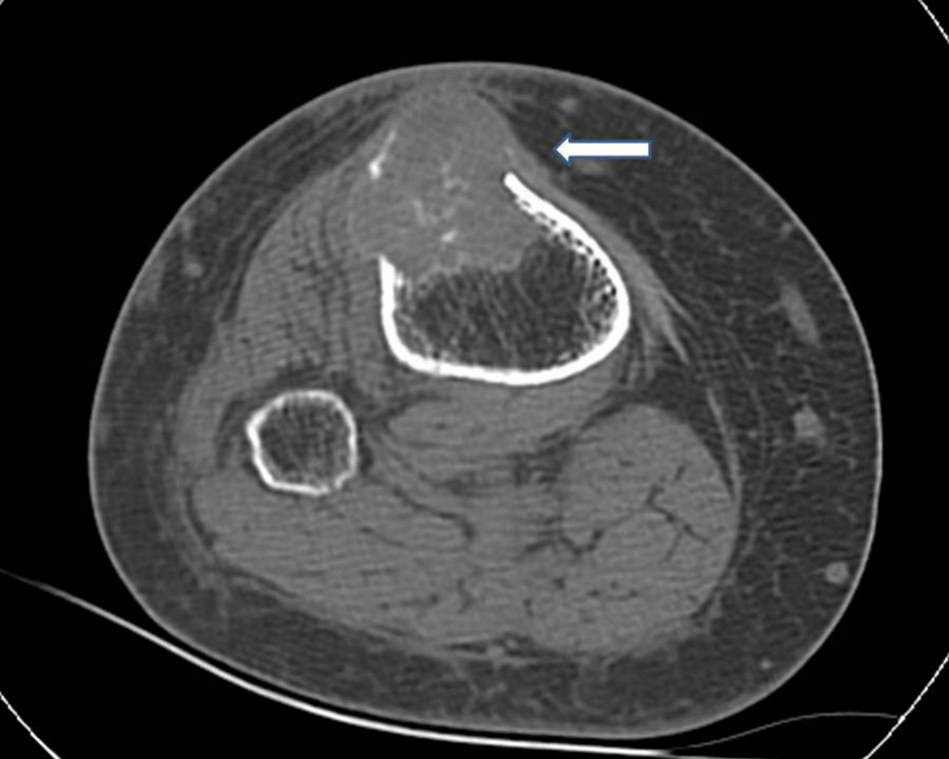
Case 3: CT scan of left proximal tibia: tumor-like extension of heterogenous soft tissue mass penetrating outside the bone (arrow)
